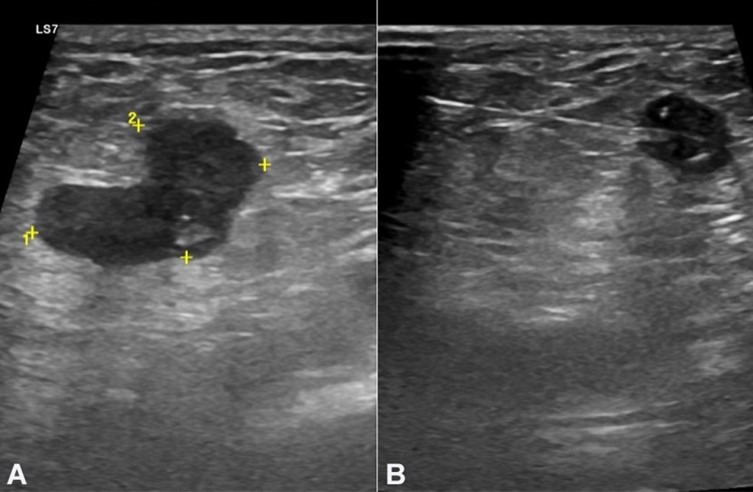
Inguinal ultrasound. Suspicious right inguinal adenomegaly measuring 20 x 11 mm (A) and the adenomegaly during the tru-cut biopsy (B).
